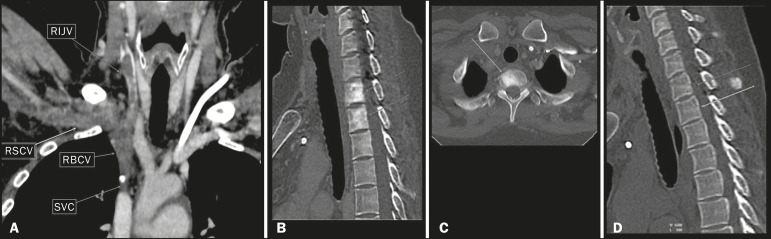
A 34-year-old female patient who was receiving peripheral parenteral nutrition after massive enterectomy, because of mesenteric ischemia three years prior, and evolved to mild pain in the cervical and right supraclavicular regions. A: CT angiography showing thrombosis of the superior vena cava (SVC). B,C: In the images that include the thoracic spine, hyperdense areas can be seen in the vertebral bodies of the upper thoracic segment. D: On an unenhanced follow-up CT angiography image, no such hyperdense areas were seen. RIJV, right internal jugular vein; RSCV, right subclavian vein; RBCV, right brachiocephalic vein.
